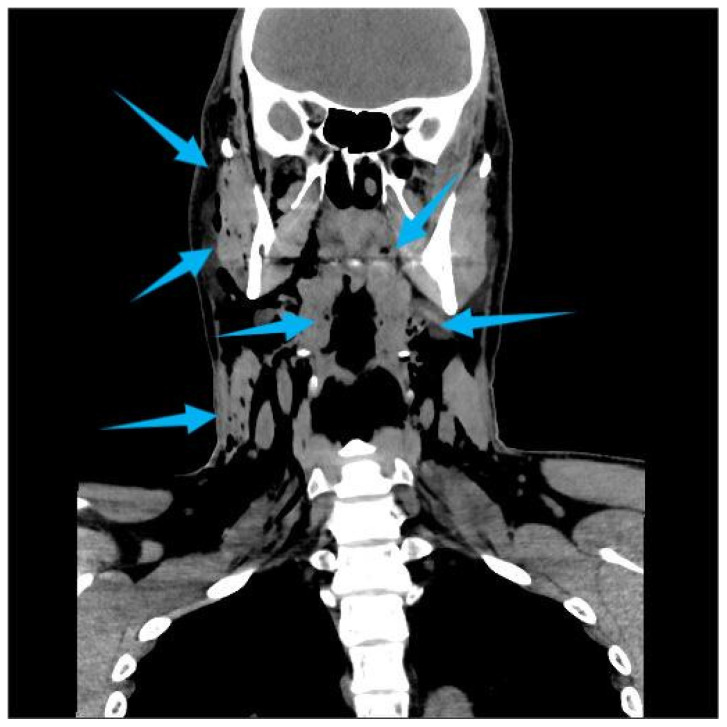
Coronal Multislice Computed Tomography (MSCT) image demonstrating extensive subcutaneous emphysema of the right side of the face, involving cervical soft tissues bilaterally and extending bilaterally into the upper thoracic region. The arrows highlight the presence of emphysema.
